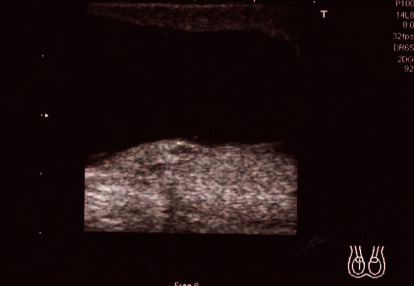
Scrotal ultrasonography image showing liquid component. Neoplastic lesions, normal testicle components, and the epididymis were not clearly visible.
